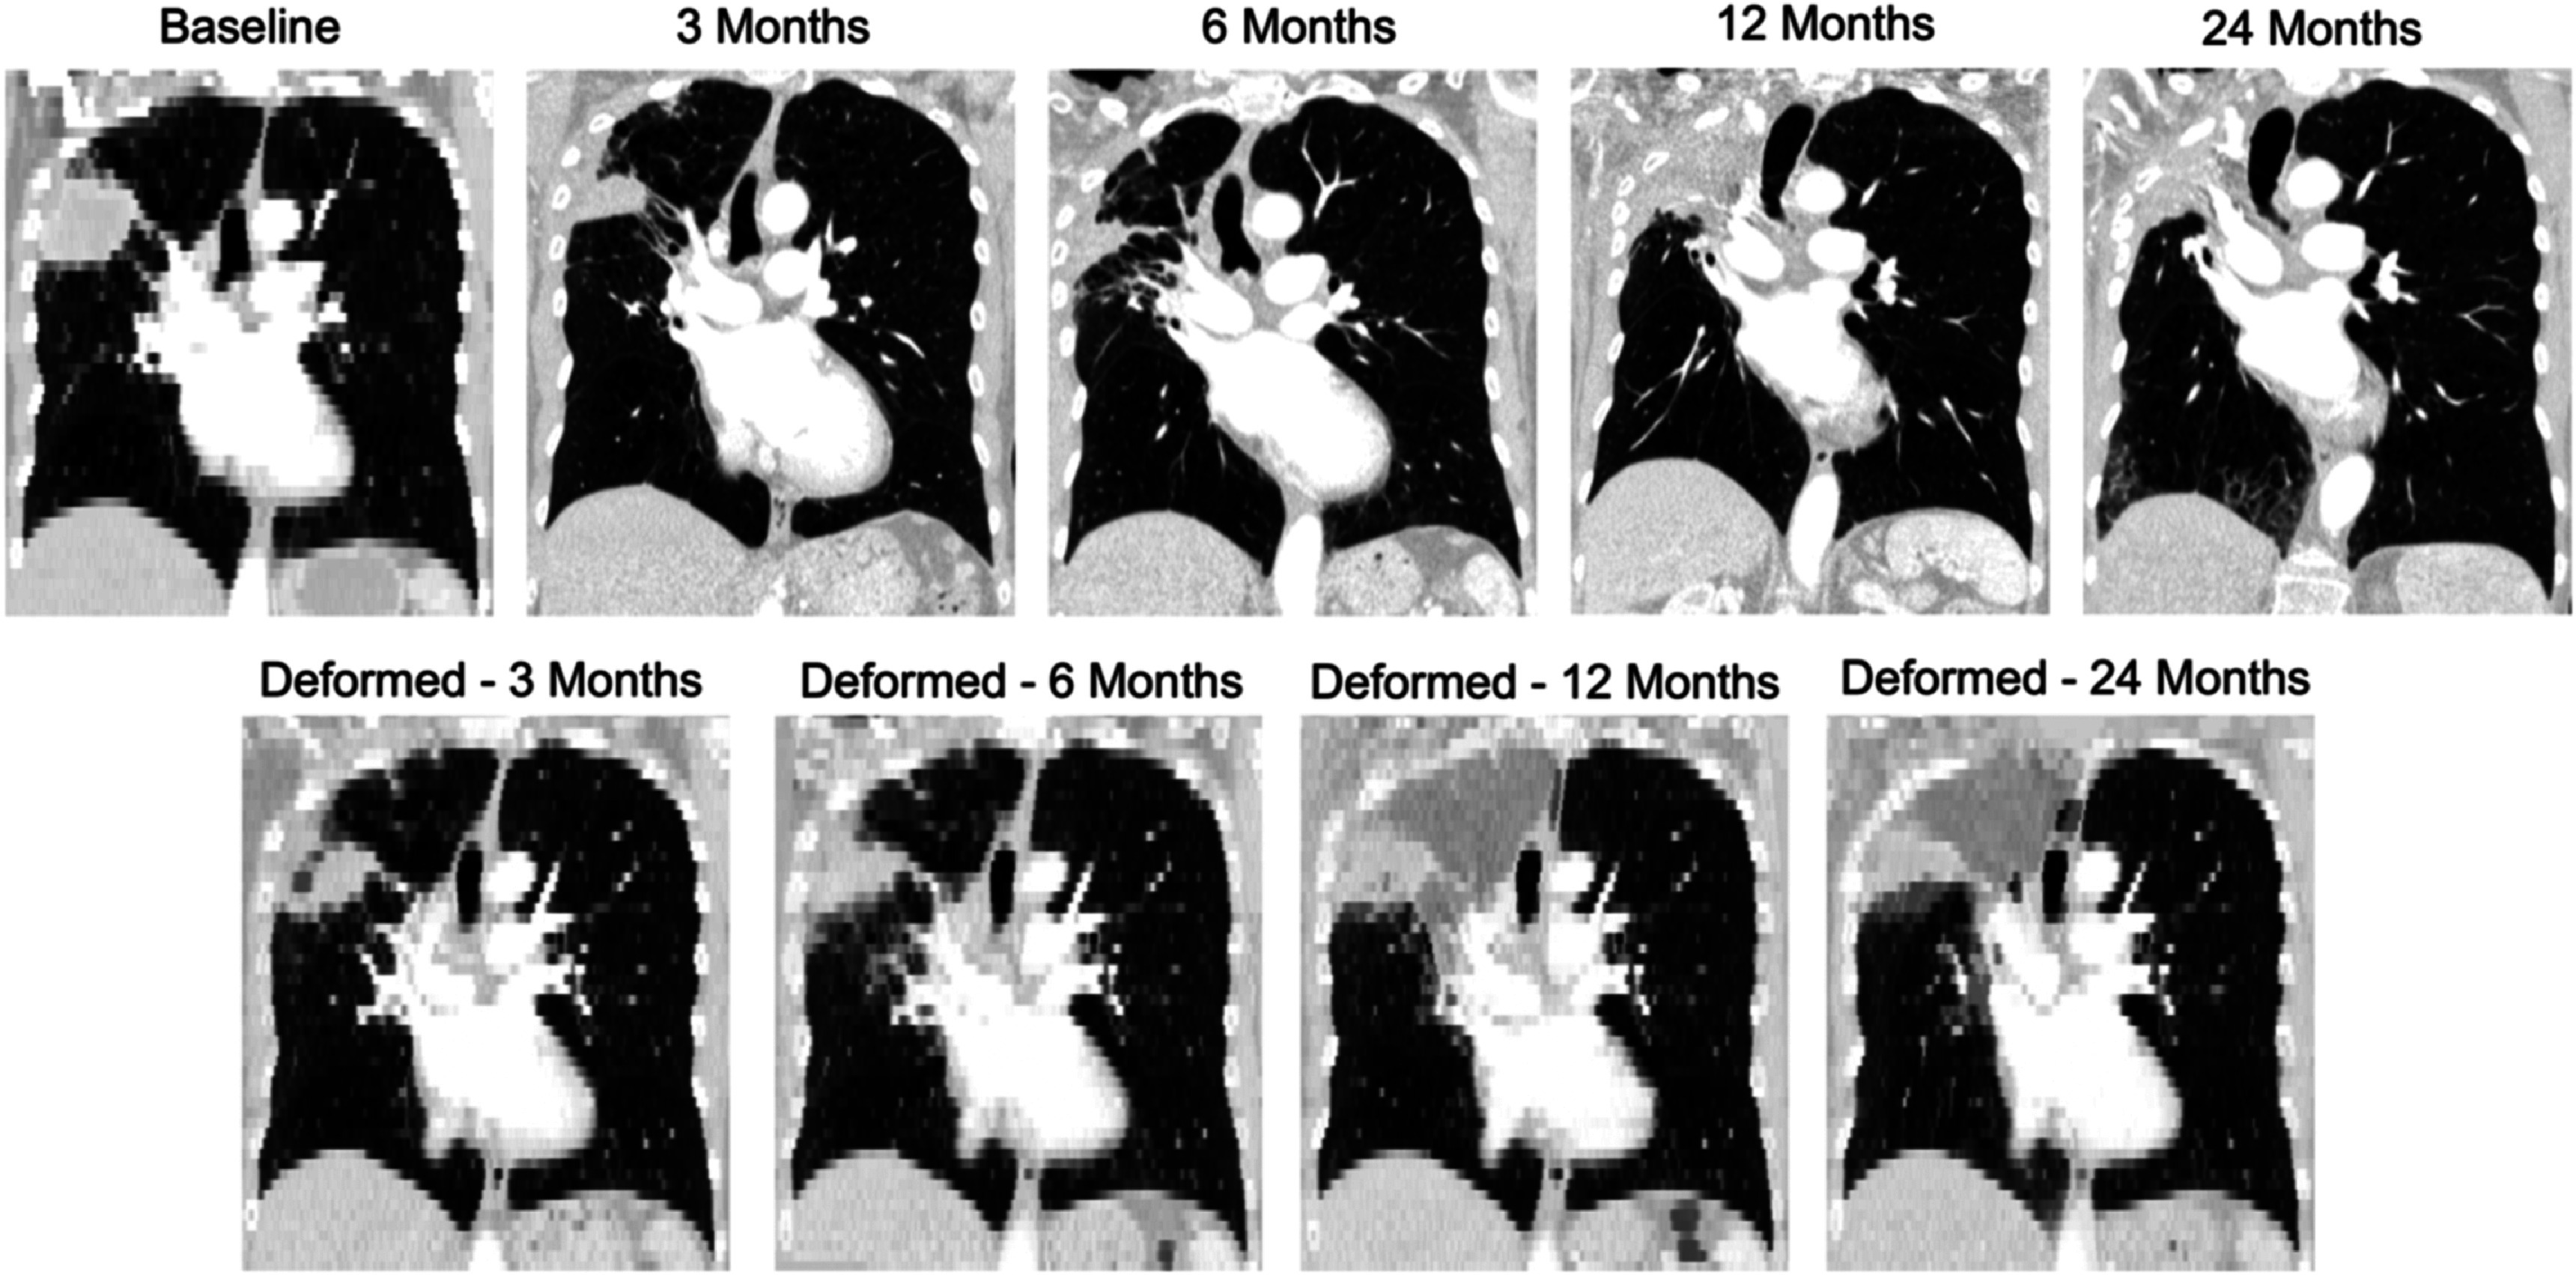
Top row: patient 15 at five time points. Upper right lobe collapse first appears in 12 month scan and remains in the 24 month scan. At 24 months new texture appears in the lower right lobe. Bottom row: colour overlays between baseline CT and registration results from registering all time points.
